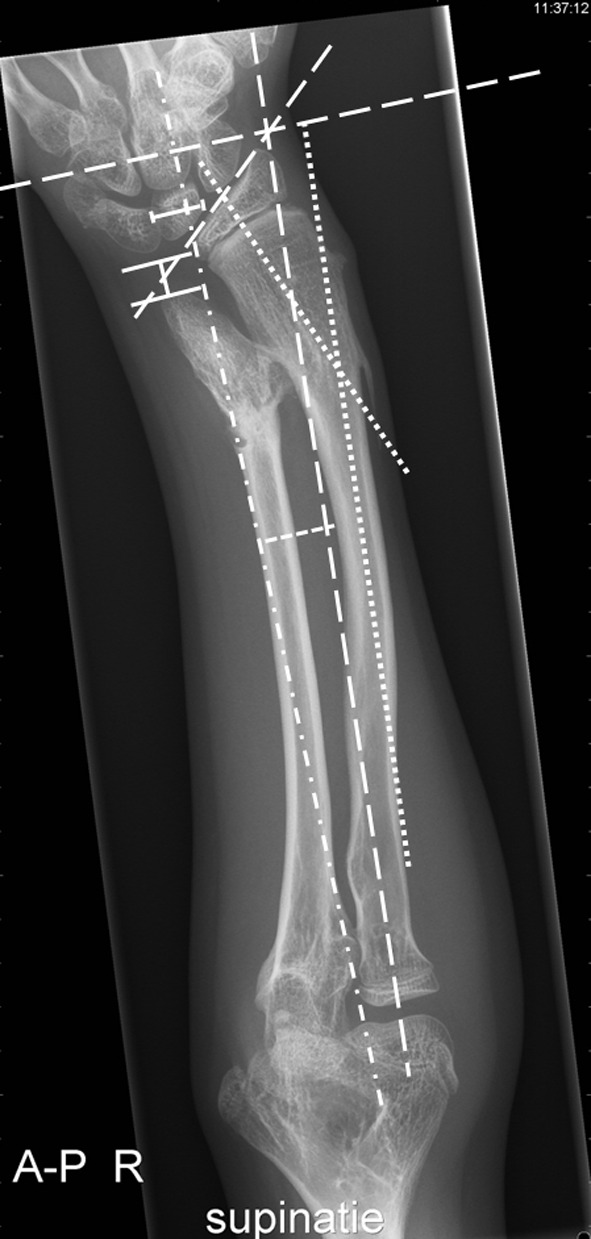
AP X-ray of the right forearm in a 14-year-old patient from the OLVG MO forearm database with Masada type I deformity: measurement of the RAA, CS, UV, RB and CORA [13]. *Dashed dotted lines* radial articular angle (RAA) between (1) a line perpendicular to a line that bisects the head of the radius and passes through the radial edge of the distal radial epiphysis and (2) a line along the articular surface of the distal radius. Normal values are defined between 15° and 30° by Fogel et al. [1]. Value measured: 43.1°.  Carpal slip (CS) percentage of the lunate surface in contact with the radius, as limited by the axial line drawn from the ulnar edge of the radial head through the ulnar edge of the radial epiphysis. This line normally bisects the lunate. Normal values for CS are >50 %. Value measured: 38 %.  Ulnar variance (UV) distance between the distal end of the ulna to the ulnar border of the distal radial epiphysis measured along the axial line. Normal values <15 mm. Value measured: 8.8 mm. *Dashed lines* radial bowing (RB) greatest distance between the radial diaphysis and the axial line. Normal values are defined as <12 mm. Value measured: 17.2 mm. *Dotted lines* centre of rotation of angulation (CORA) the intersection of the proximal axis and distal axis of a deformed bone
